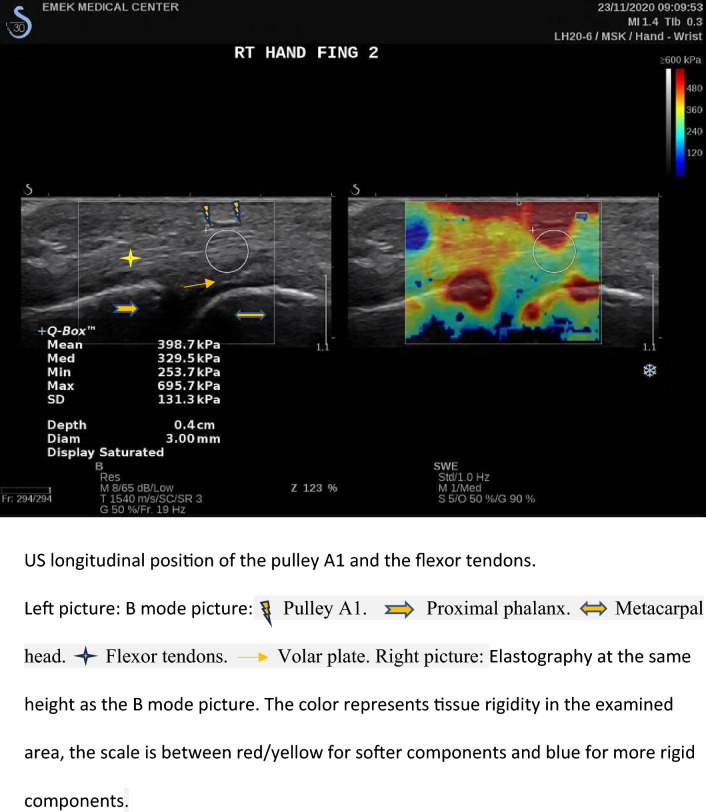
The flexor tendon in a longitudinal section below the pulley A1 in an ultrasound examination and elastography (measuring rigidity).
